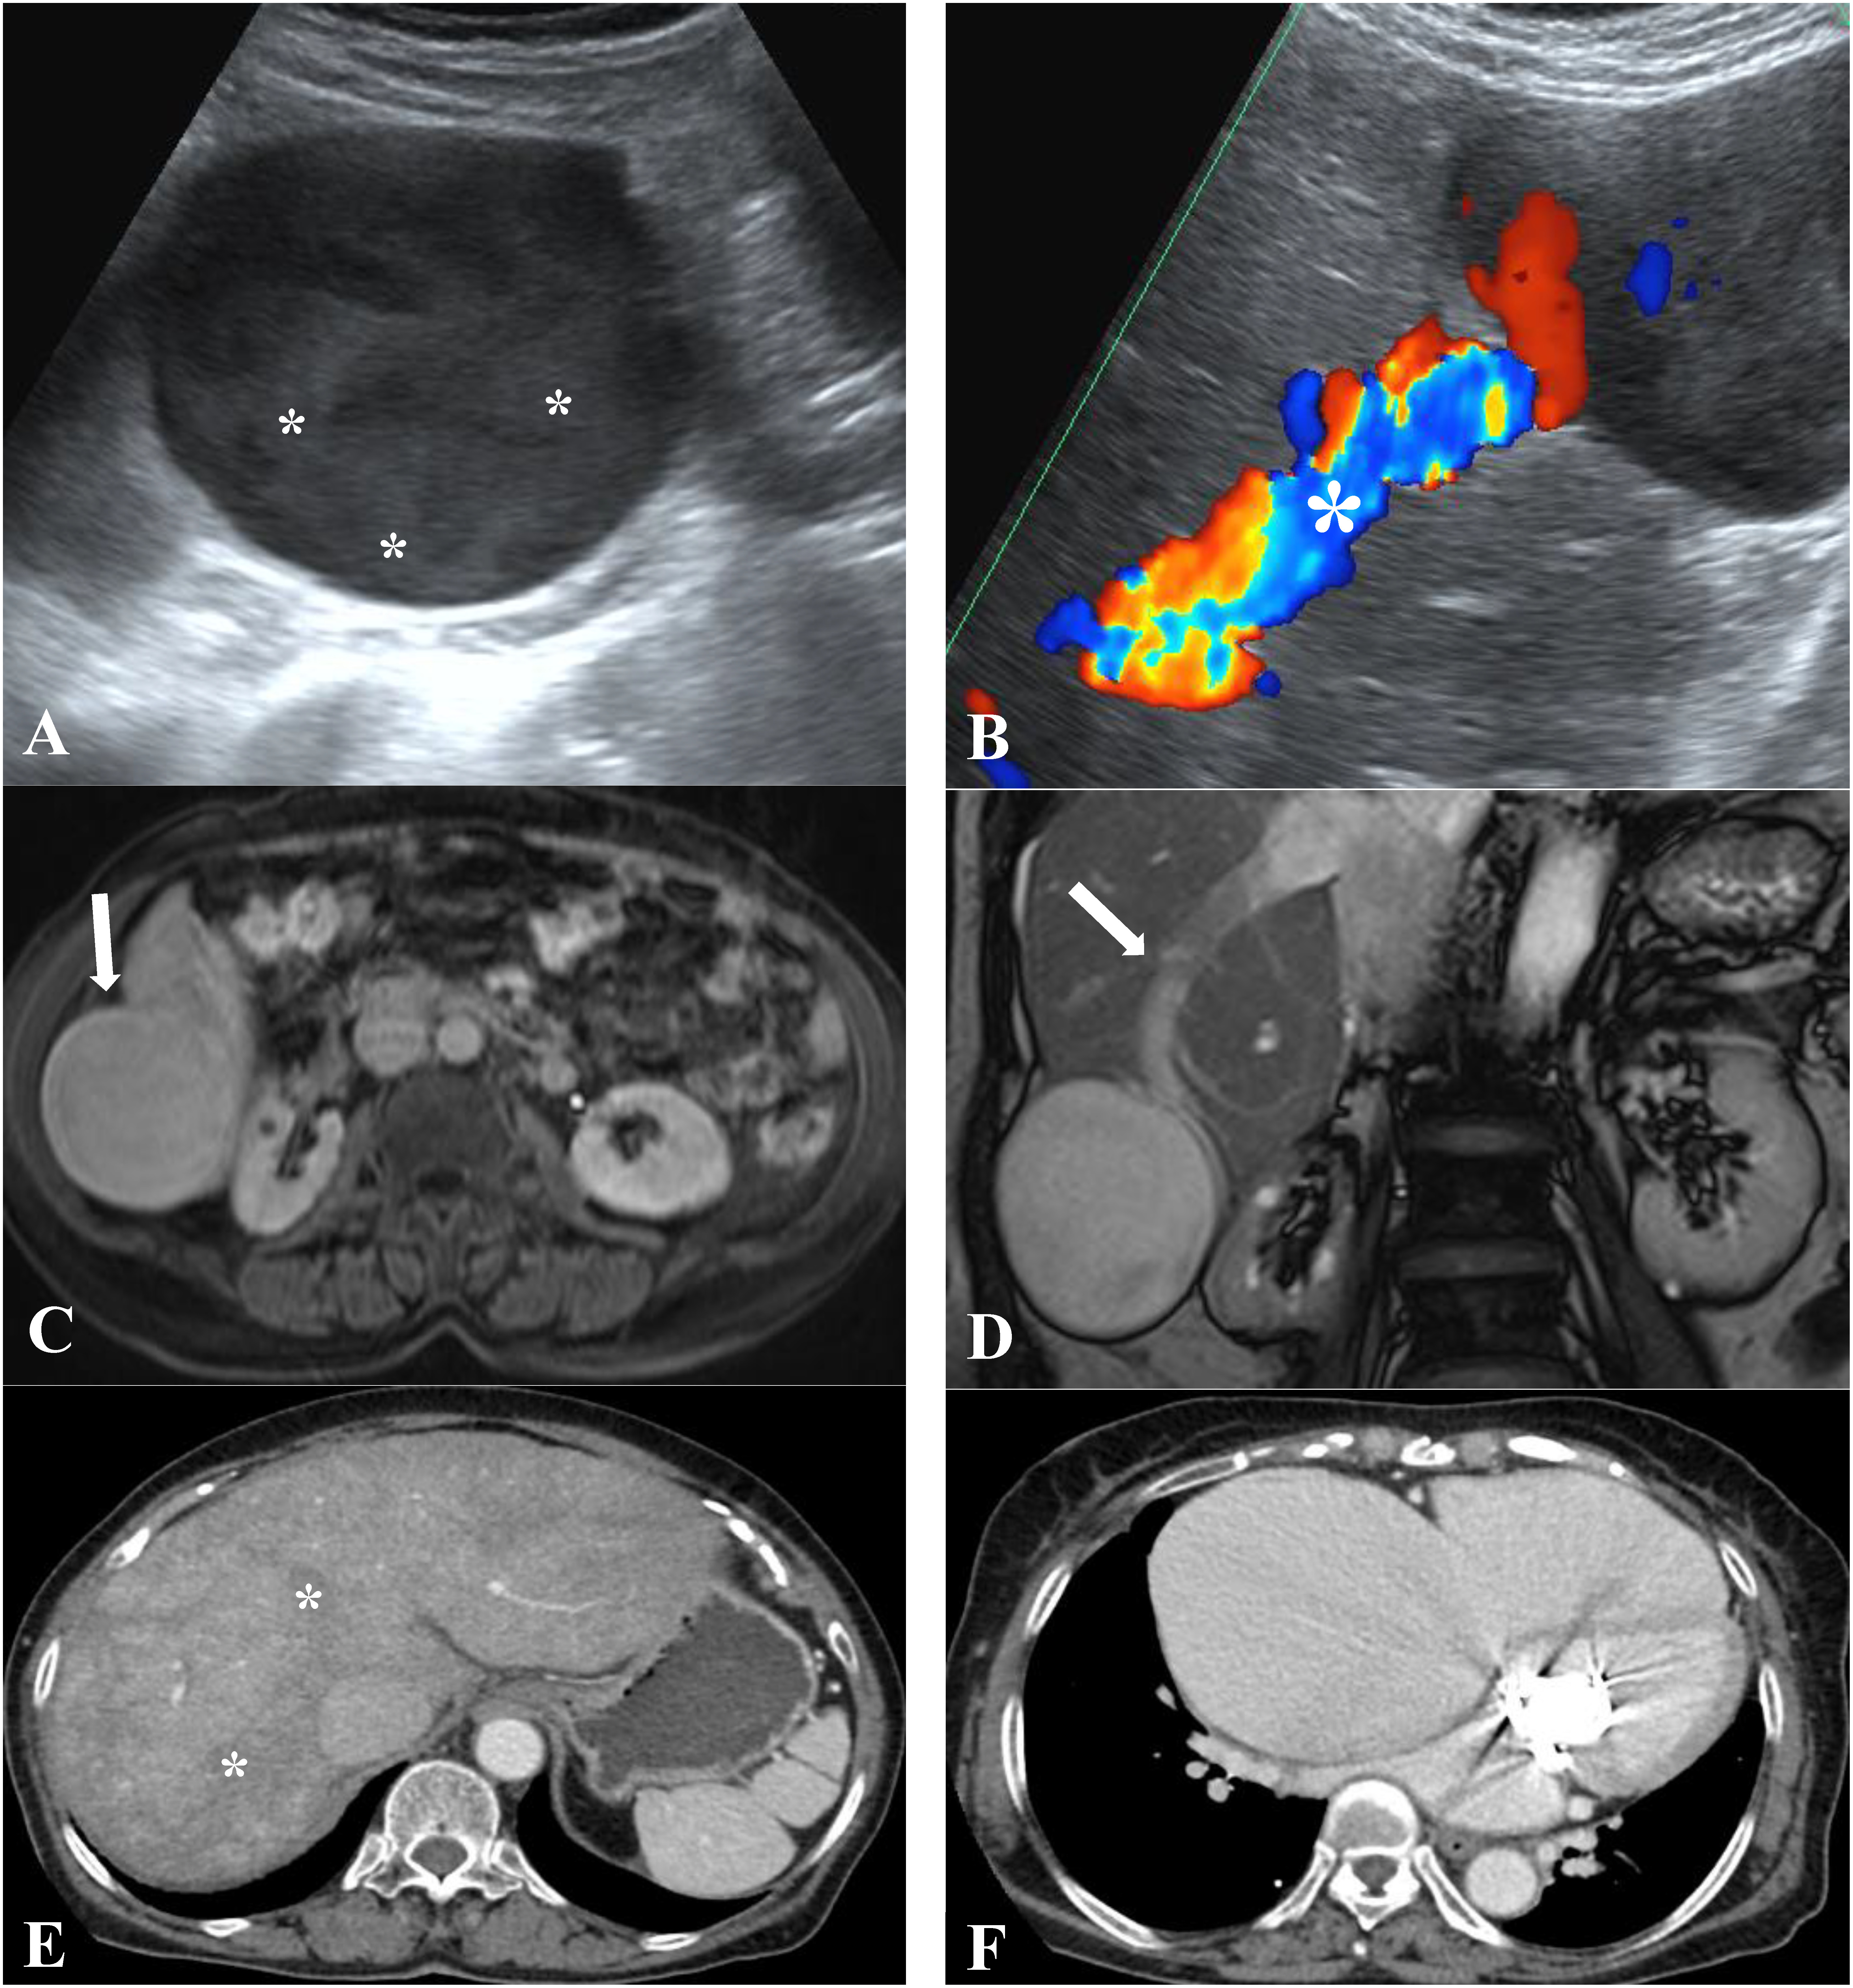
Fig. 1 (A) Grayscale ultrasound images delineate a hypoechoic lesion with suspected internal movable content (asterisks). (B) Color Doppler ultrasound images revealed a connection between the right hepatic vein (asterisk) and lesion. (C) Magnetic Resonance Imaging of the upper abdomen revealed a 6.4-cm ovoid-shaped exophytic lesion arising from hepatic segment 6 (arrow). The mass also showed delayed enhancement at 5 min after injection of gadoterate meglumine. (D) On the T2 coronal image, the connection between the hyperintensity lesion and right hepatic vein can be seen (arrow). (E) Porto-venous phase computed tomography images show a congested liver and poor enhancement of the hepatic veins (asterisks). (F) Cardiomegaly with markedly enlarged right atrium.
